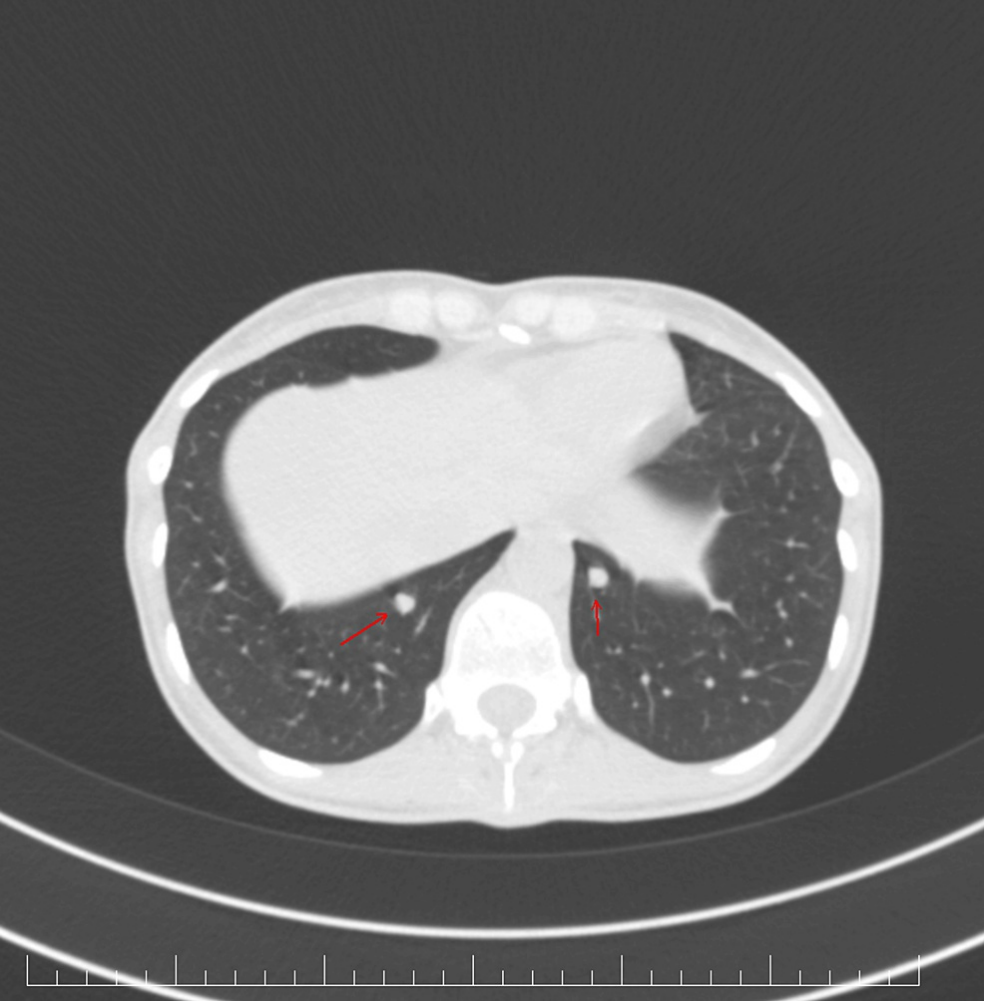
Axial view of the thoracic CT scan. Multiple pulmonary intraparenchymal nodular tissue lesions are observed, iodophile and well delimited, distributed bilaterally, predominantly at the basal regions. These lesions have a maximum diameter of 6.6 mm, noted at the level of the right Fowler segment, exhibiting suggestive features of secondary determinations.
